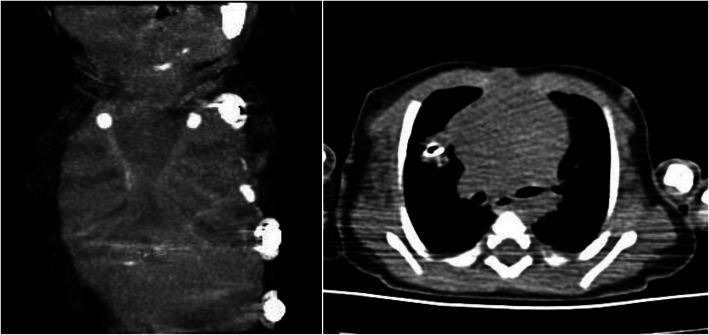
It is seen that the superior part of the sternum was undeveloped at CT. There were hypoplastic sternal bars bilaterally. Fusion defect of the superior sternum was present, and sternal bars united at the inferior
